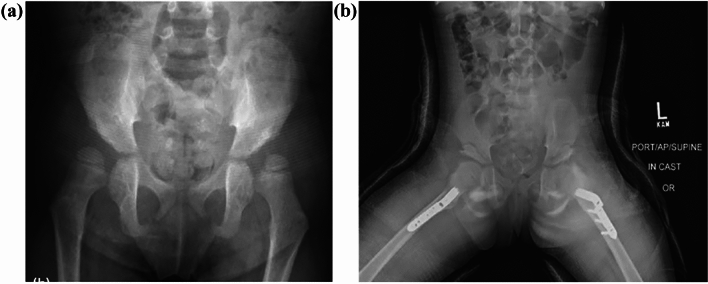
In the 2-year follow-up of the same child, bilateral acetabular dysplasia (a) is seen on anteroposterior radiograph of the pelvis. b Immediate postoperative radiograph showing bilateral Dega osteotomy and varus derotation osteotomy
